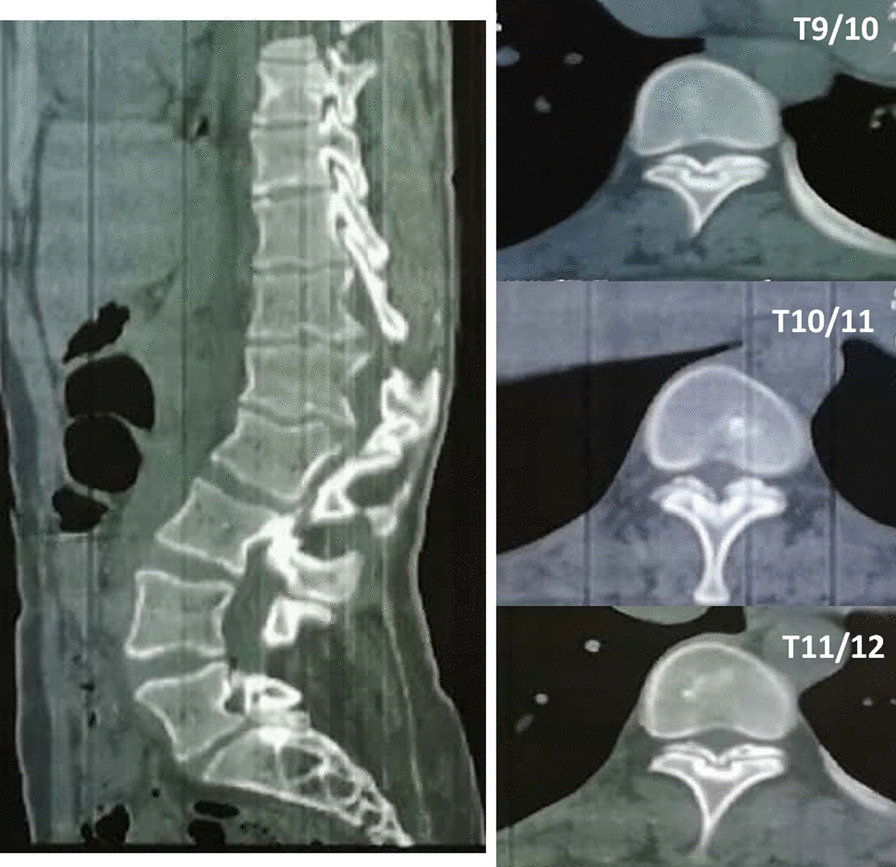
Sagittal and axial CT scans of the thoracic spine demonstrating thoracic spinal stenosis caused by multi-level ossification of ligamentum flavum and posterior osteophytes (T9/10, T10/11, T11/12)
